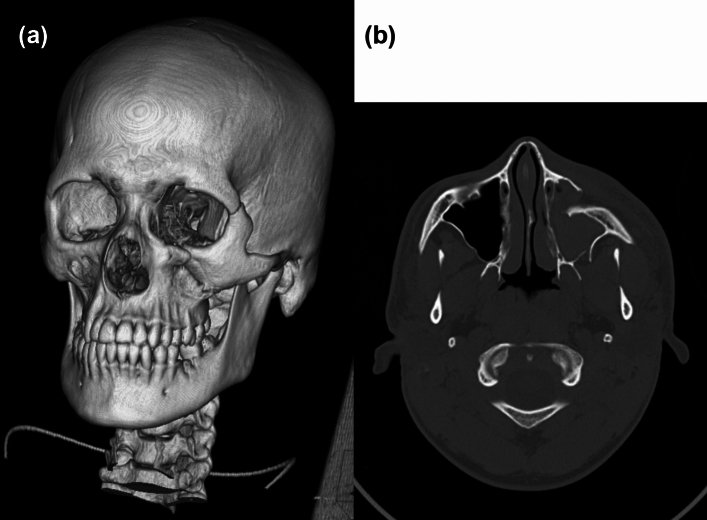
Three-dimensional volume rendering (a) and axial bone window CT image (b) of a 17-year-old player with a markedly displaced left-sided zygomatico-maxillary complex fracture
